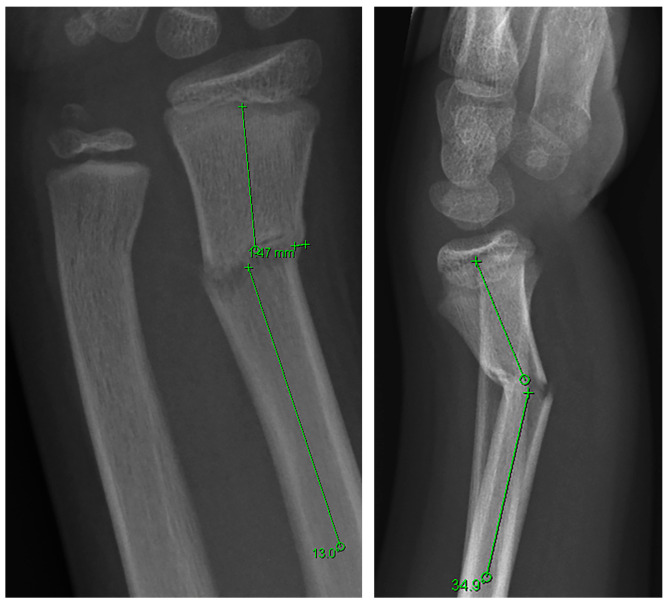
Radiographic measurement technique: Pre-reduction posteroanterior (PA) and lateral radiographs of an 11-year-old boy with a distal metaphyseal forearm fracture. The PA view demonstrates 13.0° of radial angulation of the radius, and the lateral view demonstrates 34.9° of dorsal angulation.
